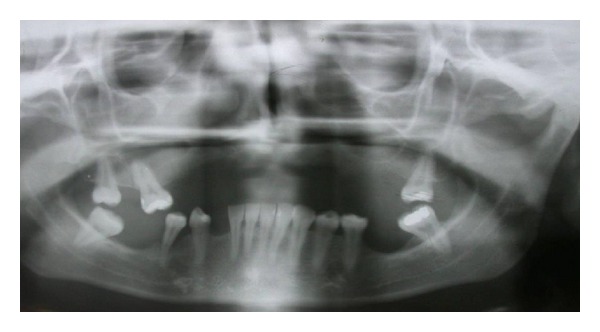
Combination Syndrome (CS) case of partially edentulous maxilla and mandible: unopposed mandibular anterior teeth are supererupted towards the atrophic premaxillary bone. Panoramic radiograph depicting anterior maxillary bone loss and showing the bone remodeling changes of the alveolar process common for CS: resorption of anterior maxilla and posterior mandible. CS classification: Class II, Mod. 3 (II-3);
